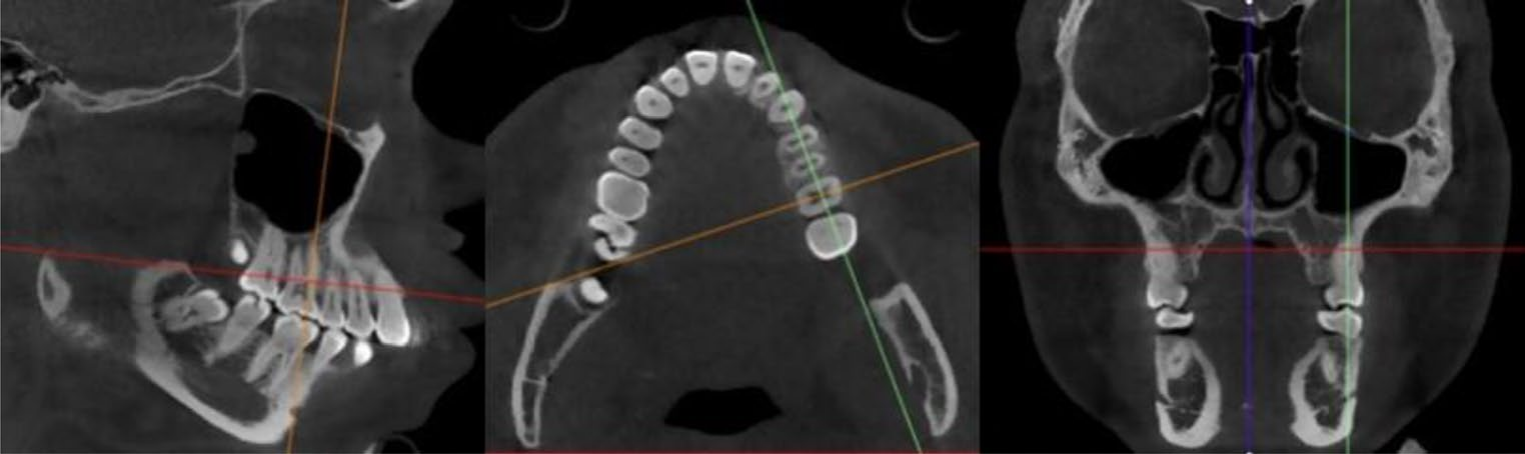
Three views of CBCT. (a) sagittal view, (b) cross-sectional, (c) axial slices
